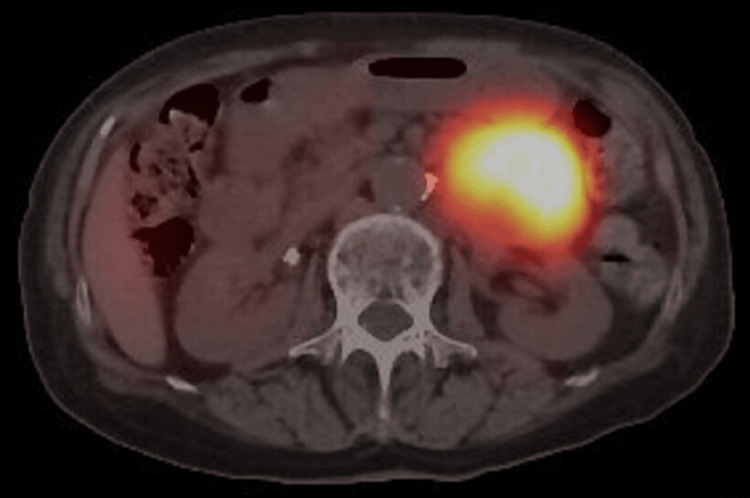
Meta-iodobenzylguanidine scintigraphy scan Pancreatic tail paraganglioma without metastasis.
